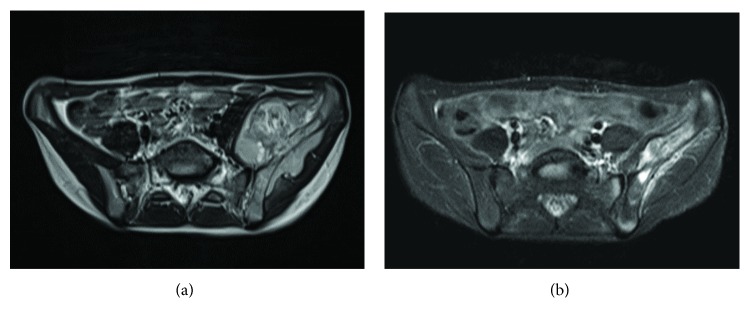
T2-weighted MRI showing a huge extraskeletal mass of pelvic Ewing sarcoma at diagnosis (a) and good response after preoperative chemotherapy (b).
